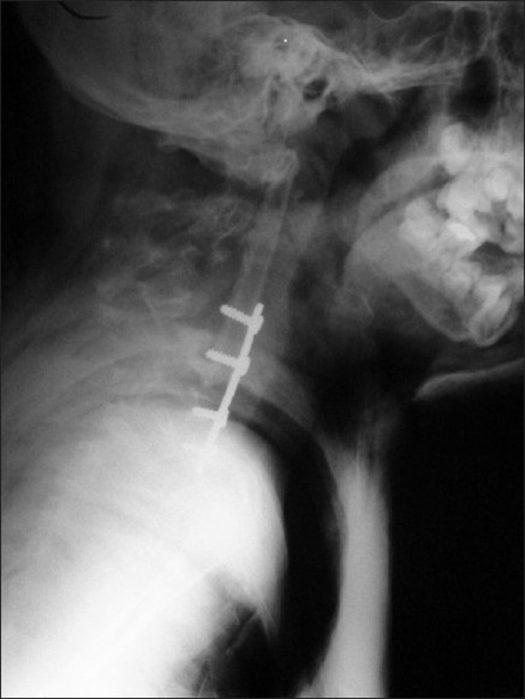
After surgery, the cast was maintained for 4 months. The radiograph show the evidence of bony healing
